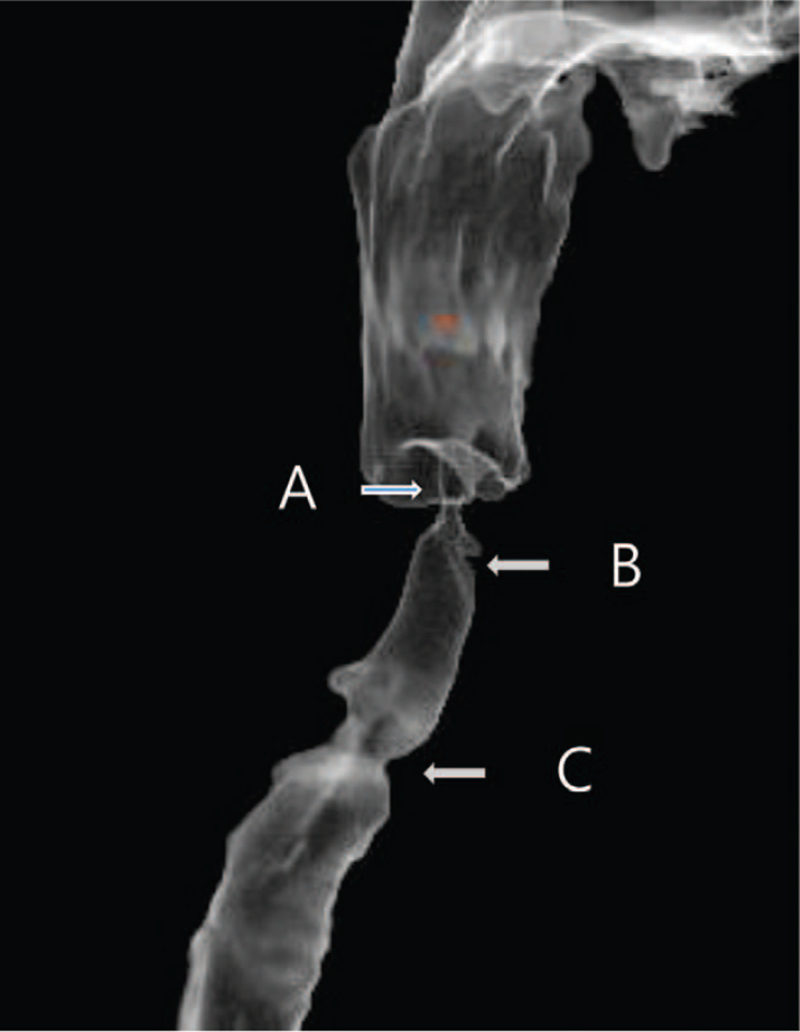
Three-dimensional reconstructed computed tomography image showing supraglottic stenosis in addition to tracheal narrowing during a previous tracheotomy. (A). supraglottic stenosis. (B). Glottis. (C). Prior tracheotomy site.
